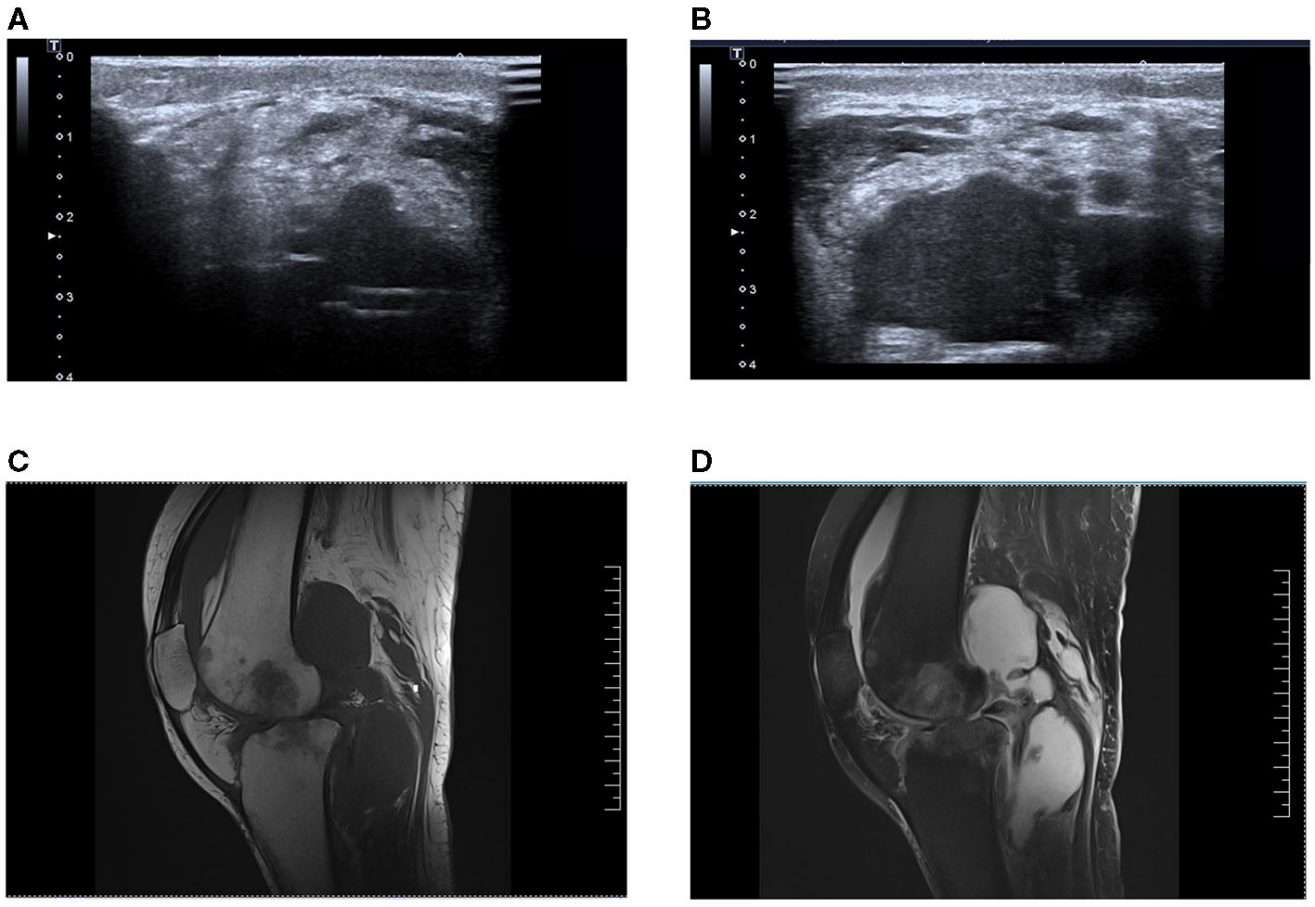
(A,B) Bedside ultrasonographic examination of knee joint cavity effusion in this patient. (C,D) Magnetic resonance imaging of the patient's knee cavity effusion, T1 image and T2 image, respectively.
